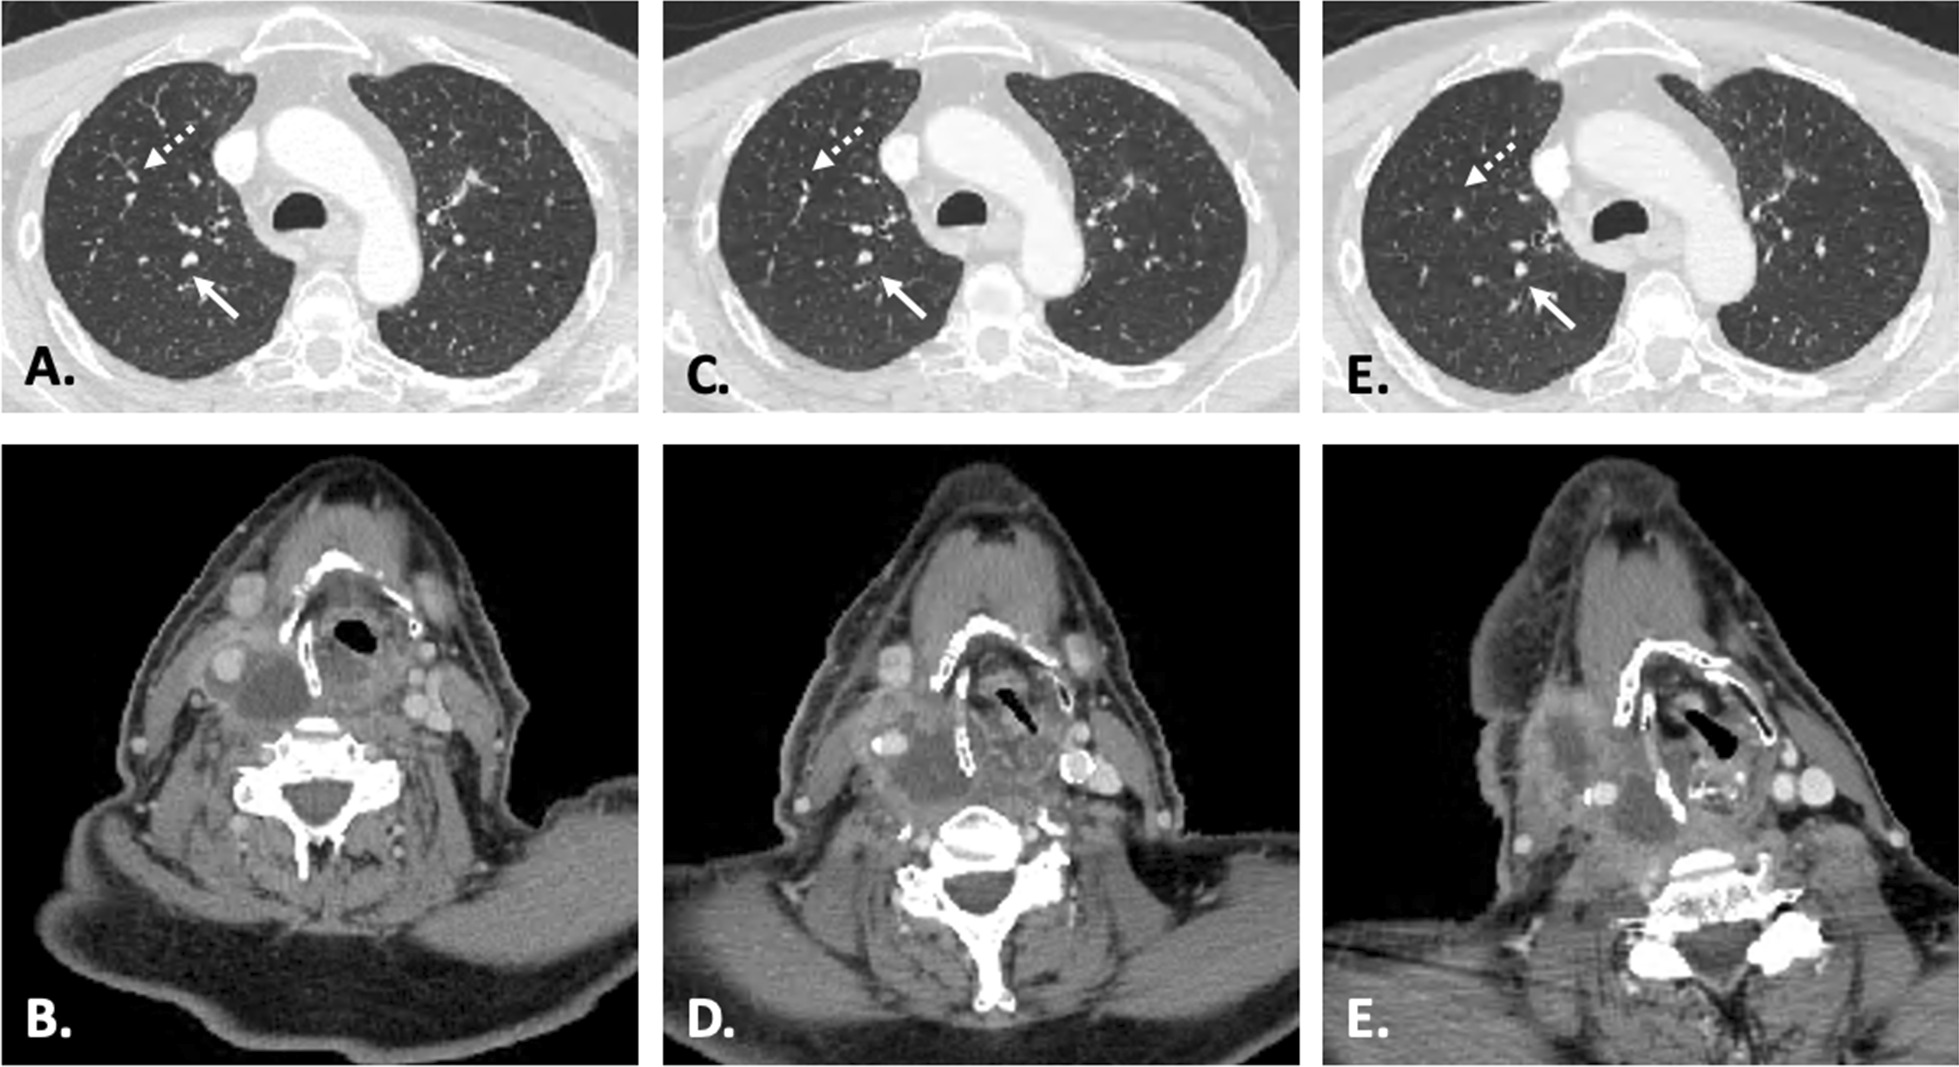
Post-immunotherapy CT imaging demonstrates enlarging thyroid tumor but stable (white arrows) or decreased pulmonary metastases (dotted arrows) after three cycles (A, B), six cycles (C, D), and nine cycles (D, E) of pembrolizumab
